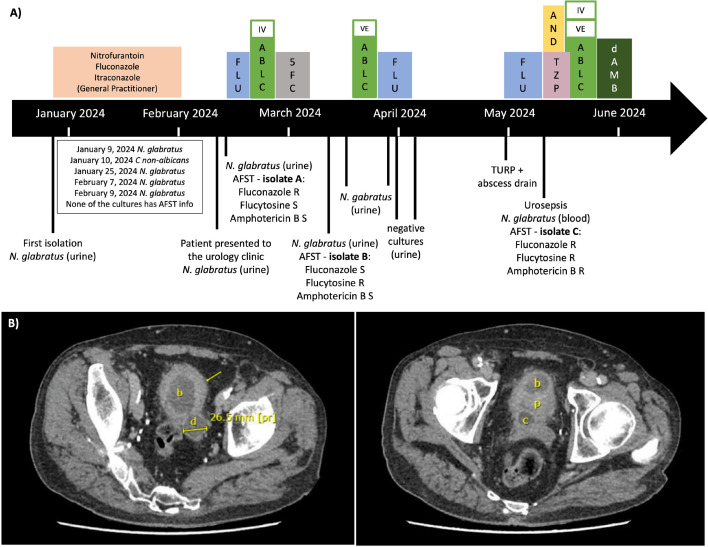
Timeline of isolation of *N. glabratus* and clinical manifestation. (A) The timeline shows the isolation of strains and antifungal susceptibility testing (AFST) data (below arrow) and treatment of the patient (above arrow). AFST details can be found in Table 1. FLU, fluconazole; ABLC, liposomal amphotericin B; VE, intravesical; IV, intravenously; 5FC, flucytosine; AND, anidulafungin; TZP, piperacillin-tazobactam; d-AMB, amphotericin B deoxycholate; TURP, transurethral resection of the prostate. AFST, antifungal susceptibility test. (B) Abdominal and pelvic CT scan in axial section with enhancement. Left image: bladder (b), bladder diverticulum (d), and thickened bladder wall (arrow). Right image: bladder (b), prostate (p), and cystic structure (c).
